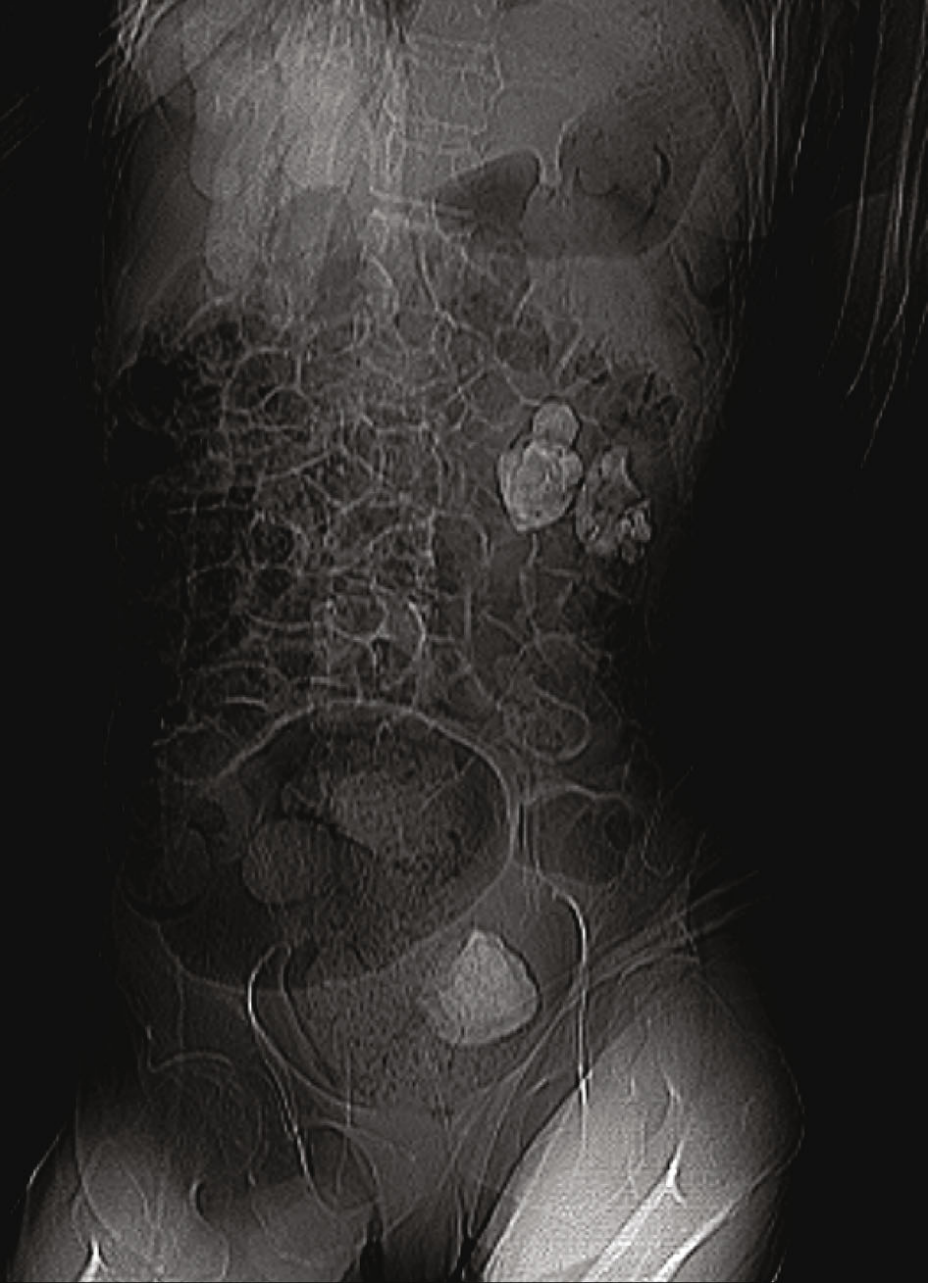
Scout film derived from the repeat CT KUB scan, revealing a staghorn calculus within the rectum, along with several large calculi inside the left kidney.
